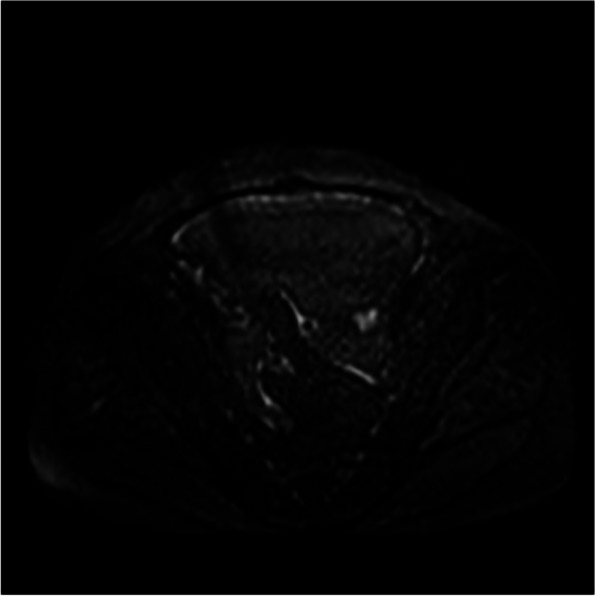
DWI tumor and peritoneum clearly shown against low signal
